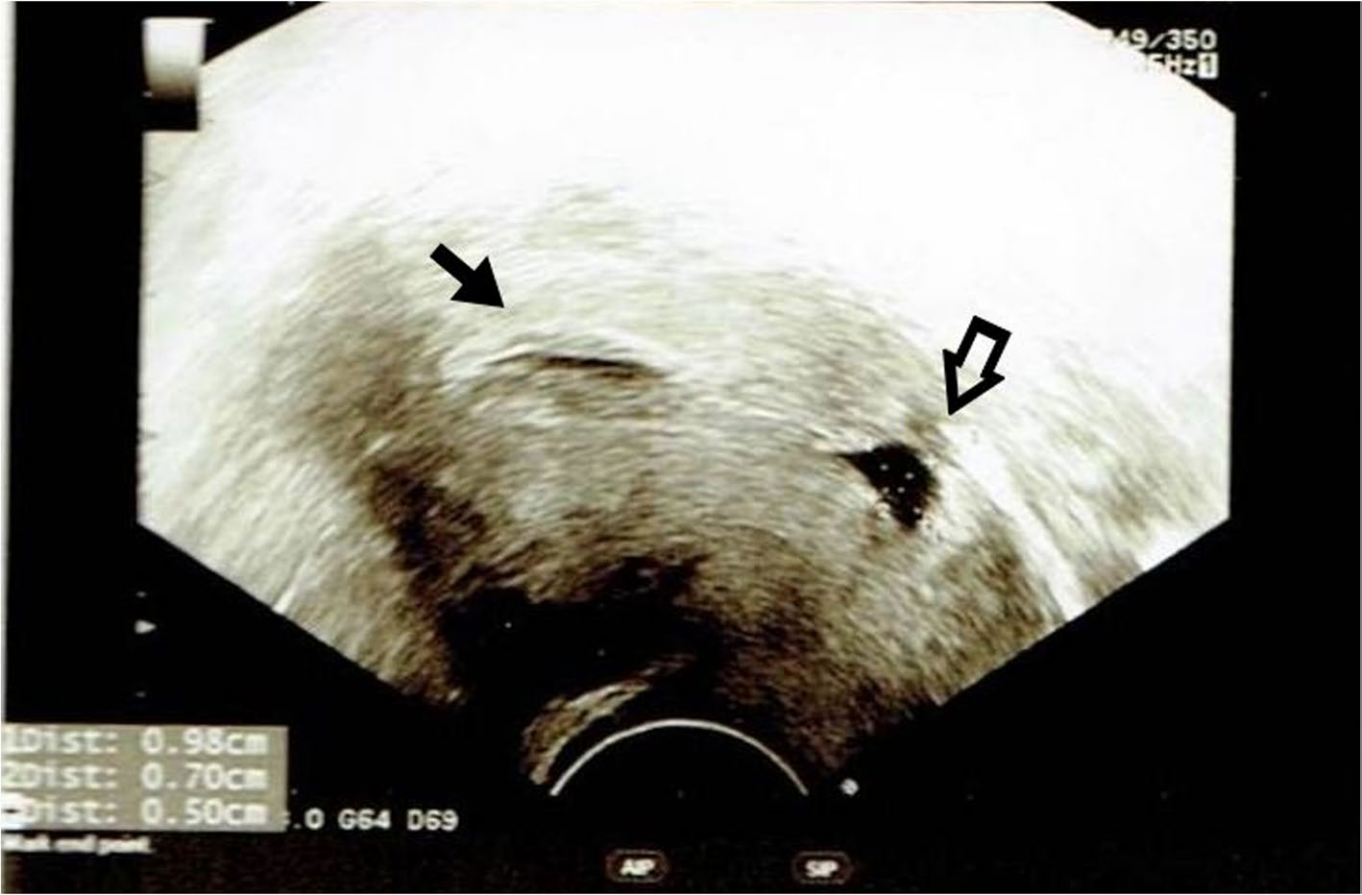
An ultrasonographic picture showing a sagittal view of the uterus and cervix. The left arrow indicates fluid accumulation in the uterine cavity, and the right arrow indicates a lesion measuring 9.8 × 7.0 mm at the lower uterine segment - a Caesarean section scar defect. A remaining myometrium thickness of 5 mm was measured
